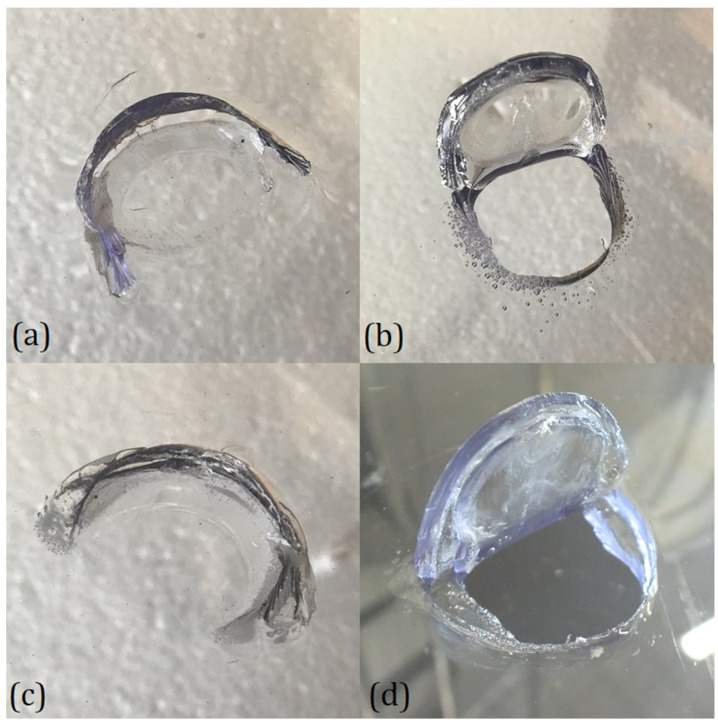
Comparison of the indentation morphologies for DWI tests: (a) contained PC, (b) uncontained PC, (c) contained LPC, and (d) uncontained LPC.
